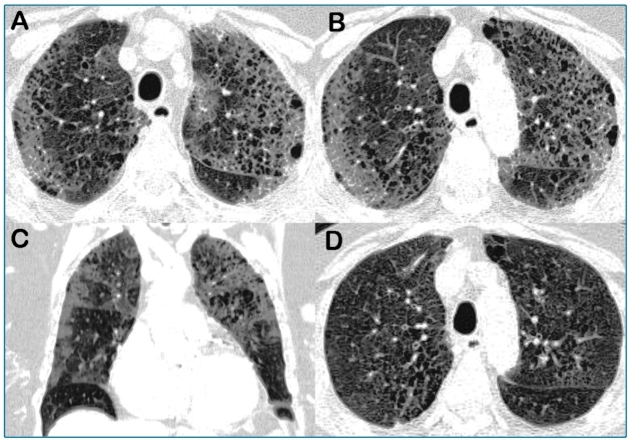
Chest computed tomography (CT) images with axial (A, B) and (C) coronal reconstruction shows small cystic formations sharing walls and ground-glass opacities predominating in the upper lobes. This pattern was suggestive of honeycombing. (D) A chest CT examination performed one year previously demonstrates centrilobular emphysema in the upper lobes.
